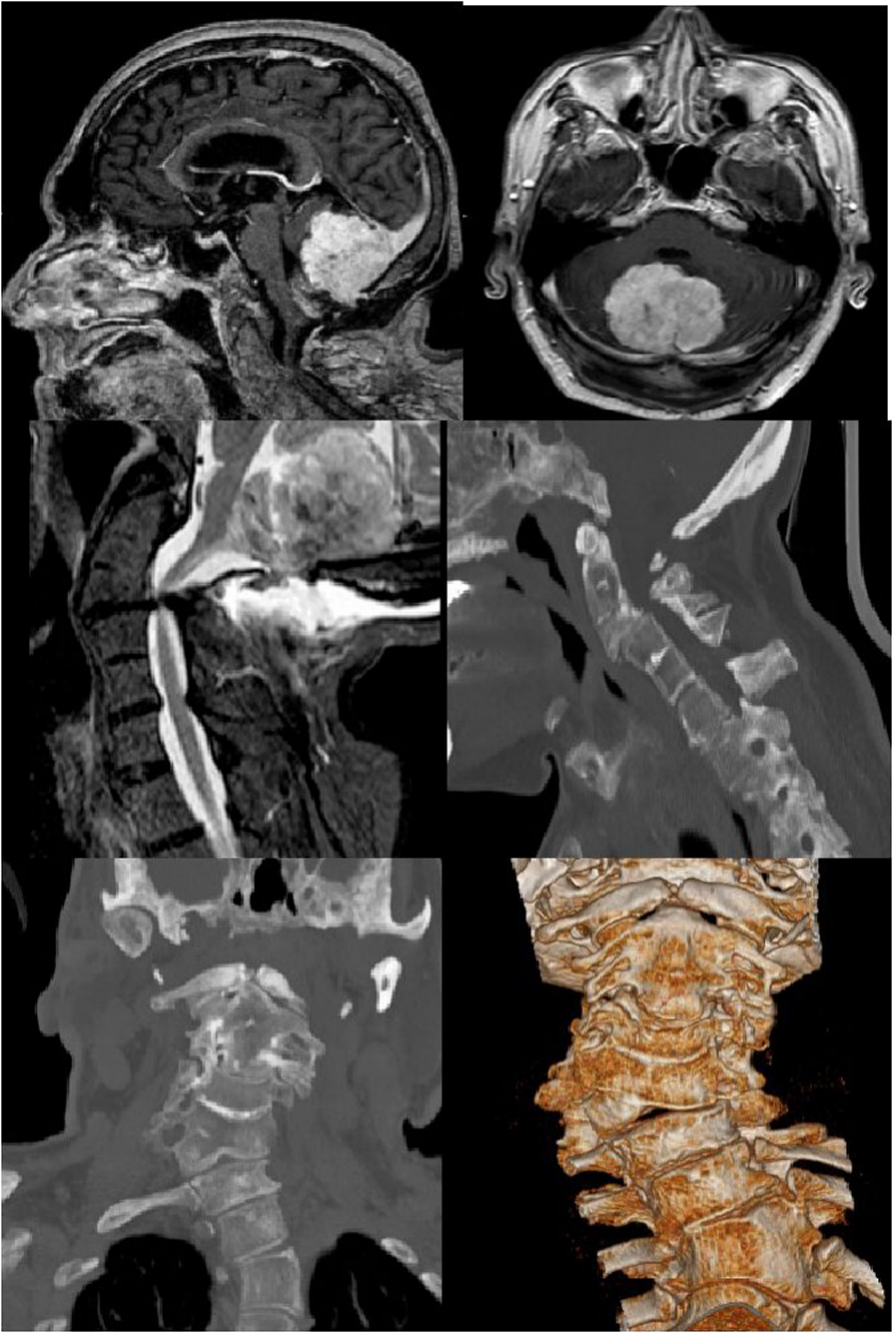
Sagittal (*upper left)* and axial (*upper right*) contrast-enhanced T1-weighted magnetic resonance imaging showing a fourth ventricle tumor with infiltrative features and non homogeneous signal. Sagittal craniocervical T2-weighted magnetic resonance imaging *(middle left*), sagittal craniocervical scanning (*middle right*) and coronal computed tomography images (*lower right and left*) revealing a scoliotic deformation of the vertebral column with C3 to C4 synostosis
